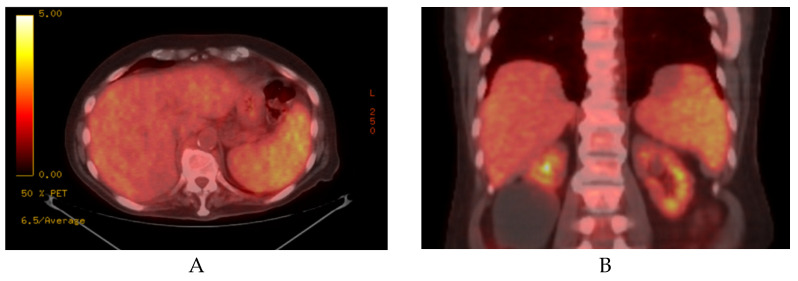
Axial (A) and coronal (B) sections of a PET/CT scan showing mild-moderate splenomegaly with increased FDG uptake in a patient with SDRPL.
